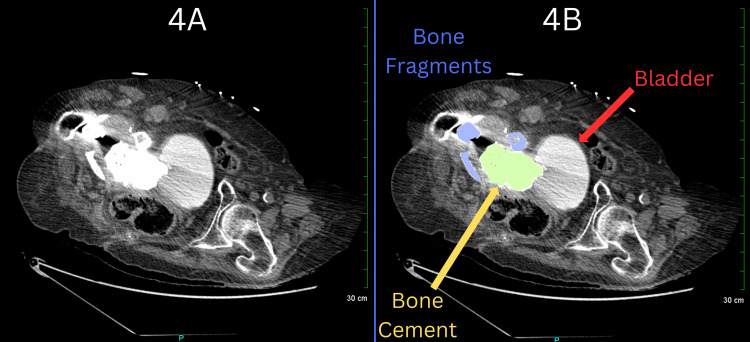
CT angiography of the pelvic region. Panel 4A displays the unlabeled image, while Panel 4B presents the labeled image for anatomical reference. The bone cement is highlighted in yellow, protruding into the bladder as indicated by the red arrow. Bone fragments, marked in blue, are visible on the right side of the body. CT: Computed tomography
